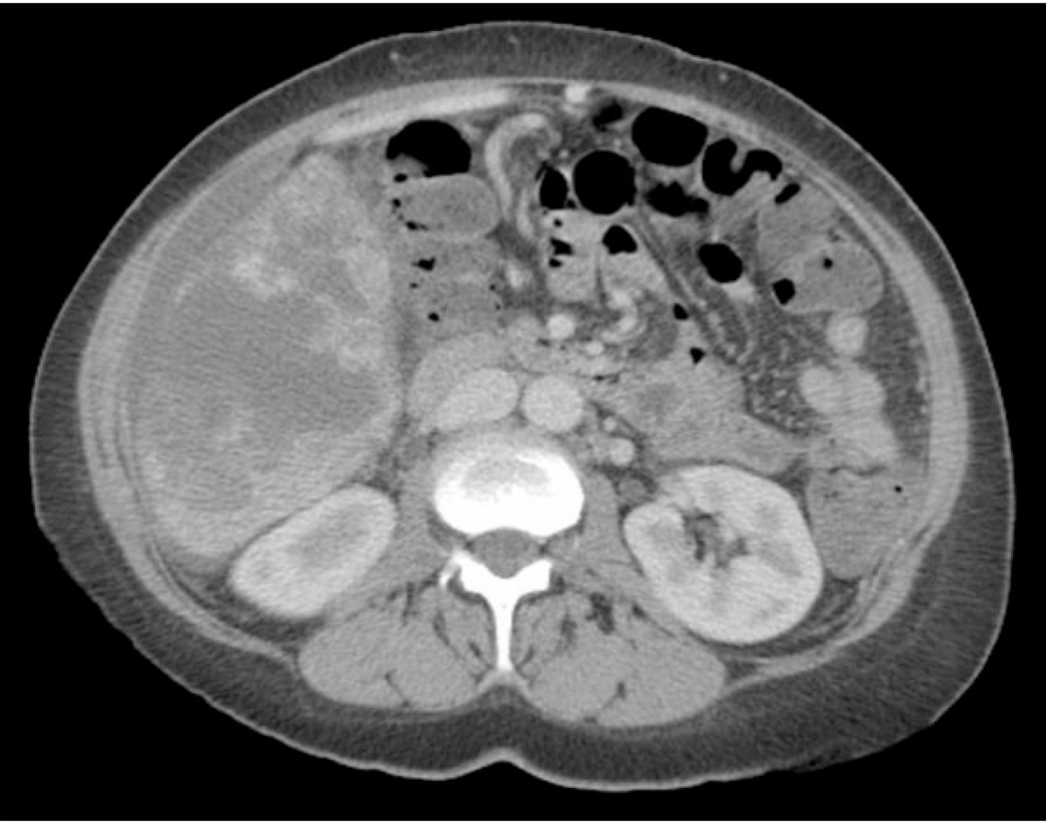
CT scan of the abdomen and pelvis with intravenous contrast showing multiple masses throughout the liver, later biopsy-proven hepatic angiosarcoma
